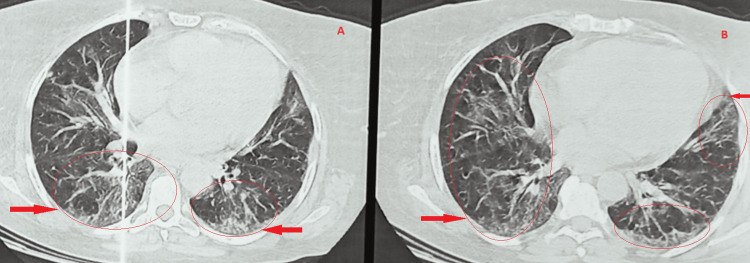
Chest CT scan performed during her hospitalization in 2022 showing a bilateral frosted-glass image and septal thickening realizing the crazy paving appearance.
